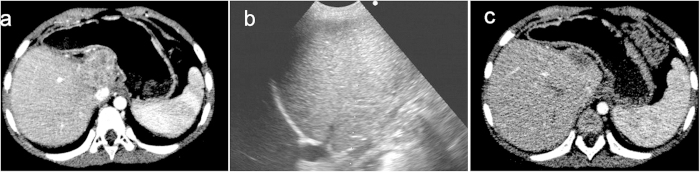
A 4-year-old girl with recurrent hepatoblastoma after initial liver resection. Contrast-enhanced computer tomography (CT) shows a 3.1 cm recurrent tumor in the caudal lobe (a). After percutaneous ethanol injection (b), contrast-enhanced CT shows disappearance of the enhancement within the treated tumor (c).
